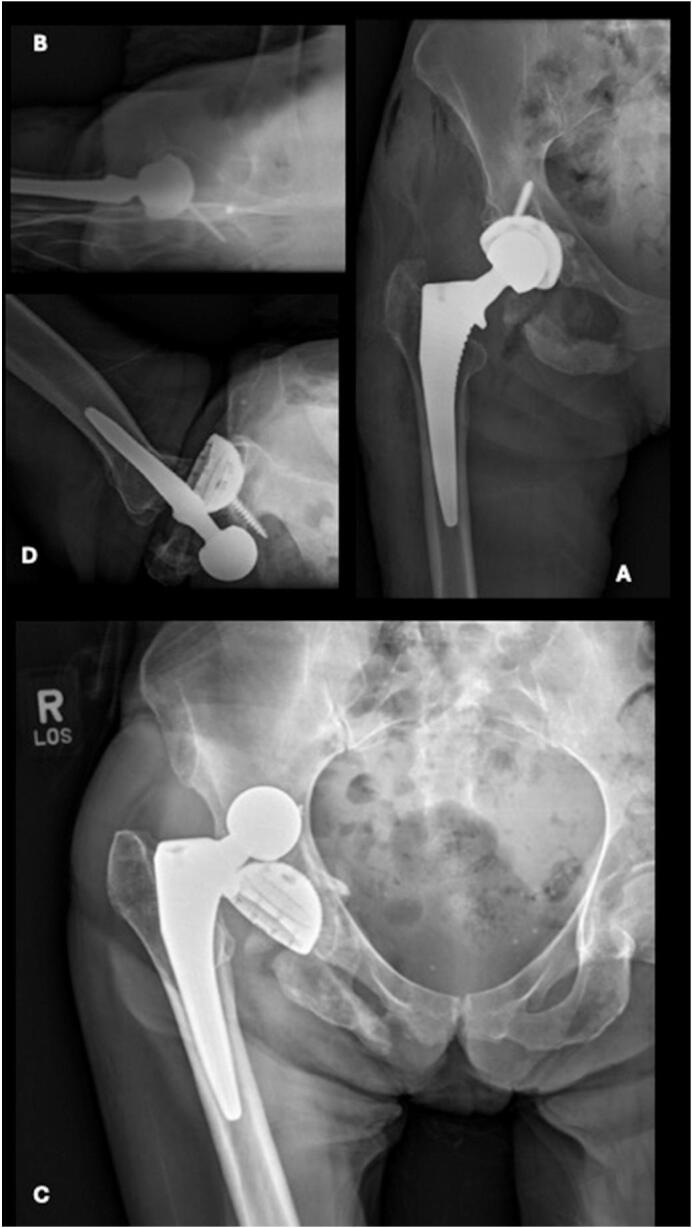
AP (A) and lateral (B) radiographic images of right hip showing concentrically reduced right total hip arthroplasty. AP (C) and lateral (D) radiographic images of right hip status post right total hip arthroplasty with posterior and superior dislocation.
